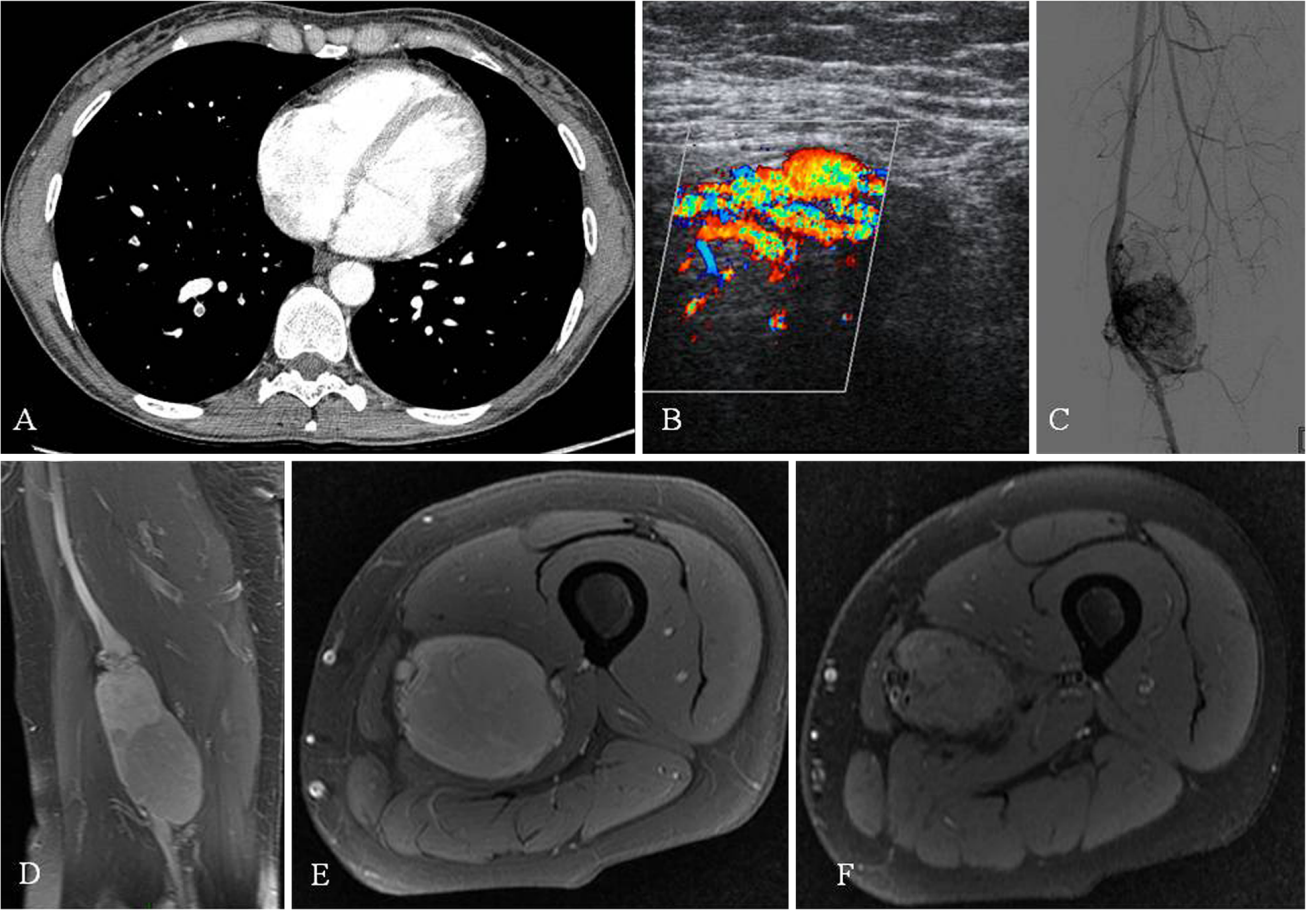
A: CT scan showing PE. B: Doppler ultrasonography showing hypervascularization of the lesion. C: Arteriography showing the location of the lesion adjacent to the superficial femoral artery and the superficial femoral vein. D,E,F: MRI of the femur showing a mass close to the superficial femoral artery and the superficial femoral vein that appears compressed and deformed.
